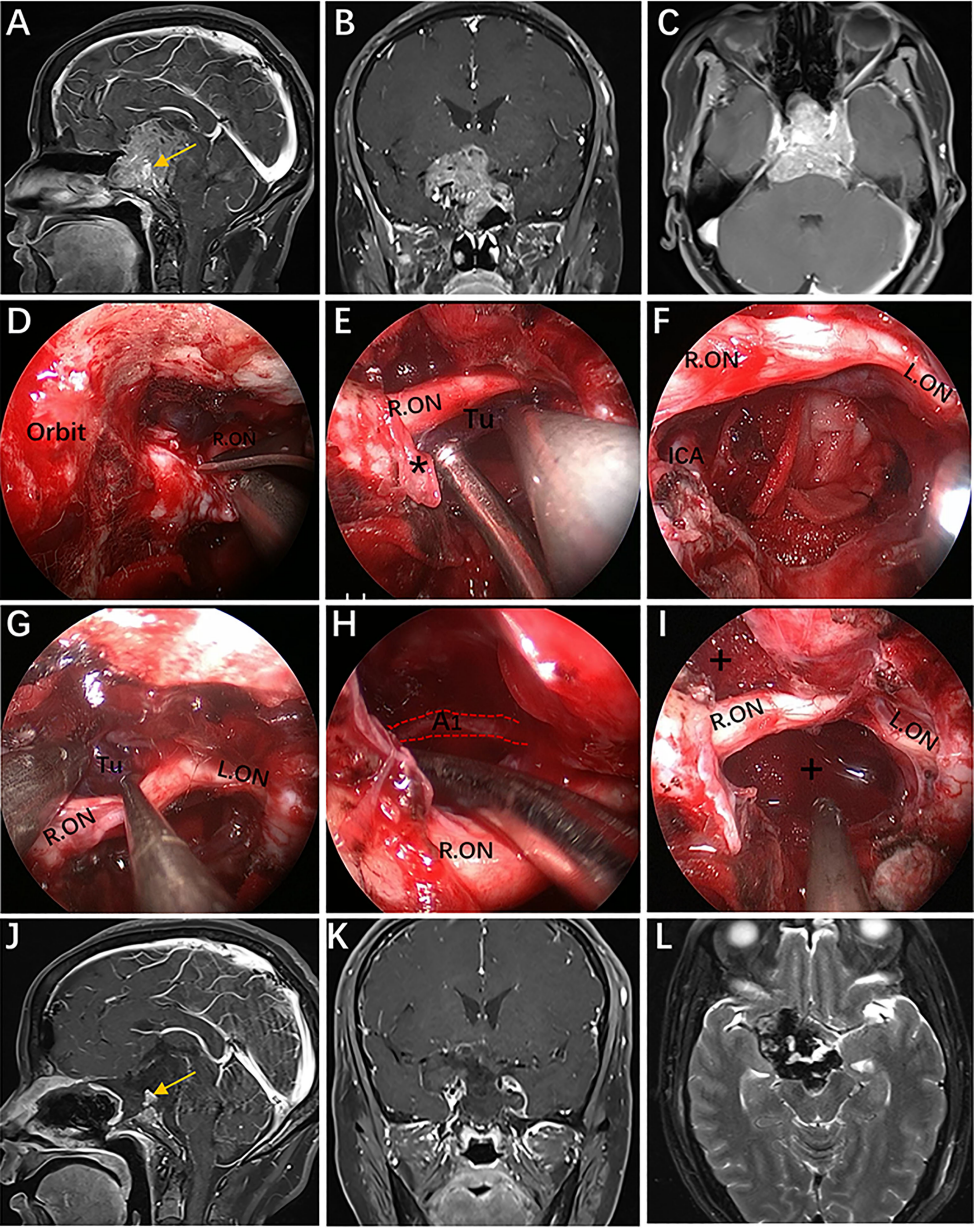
Case 6, a 45-year-old woman presented with visual loss and headache for 2 years. (A–C) Preoperative Gd-enhanced MRI showing a multilobulated pituitary adenoma with bilateral cavernous sinus invasion, inferior extension into the clivus, superior compressed the third ventricle, and significantly encasement of the ICA and its bifurcation. (D–I) Intraoperative images. (D) Opening of the optic nerve sheath. (E, F) Removal of the tumor below the right optic nerve using a bimanual microsurgical technique and endoscopic view after resection. (G) Expose the tumor above the optic nerve. (H) Removal of the tumor using angled suction instruments through the supraoptic corridor. The red dotted line indicates the course of the A1 segments of the anterior cerebral artery. (I) Endoscopic view after tumor resection. (J–L) Postoperative MRI showing total tumor removal. The yellow arrow represents the normal pituitary gland. * = optic nerve sheath; + = tumor cavity. The figure is available in color only online.
